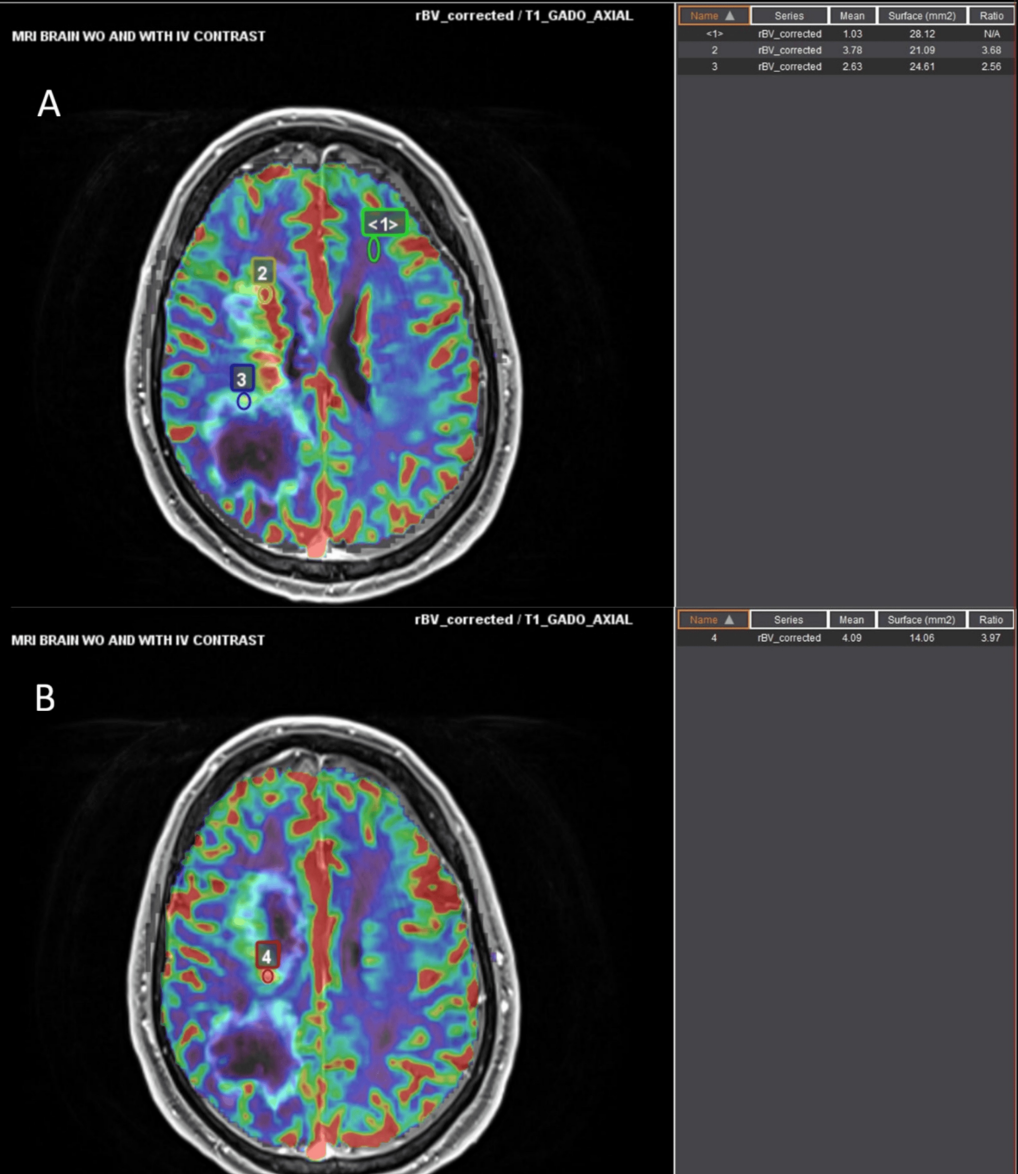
MR DSC perfusion Dynamic susceptibility contrast (DSC) MR perfusion applied to the original MRI brain demonstrated elevated relative cerebral blood volume within the enhancing margins of the lesions. Image A shows points 1-3, which correspond to relative cerebral blood volume (rCBV) values of 1.03, 3.78, and 2.63, respectively. Image B shows point 4, which corresponds to an rCBV value of 4.09.
